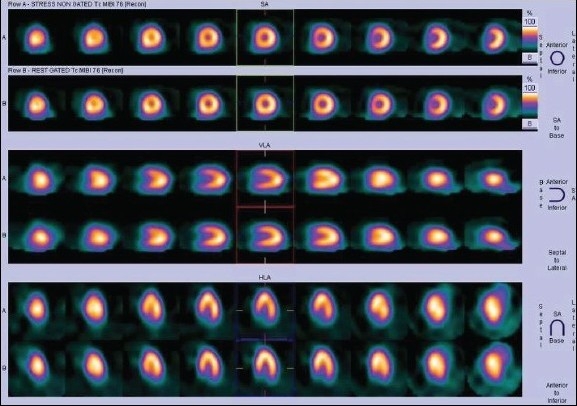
^99m^Tc MIBI normal stress myocardial perfusion single photon emission computed tomography
